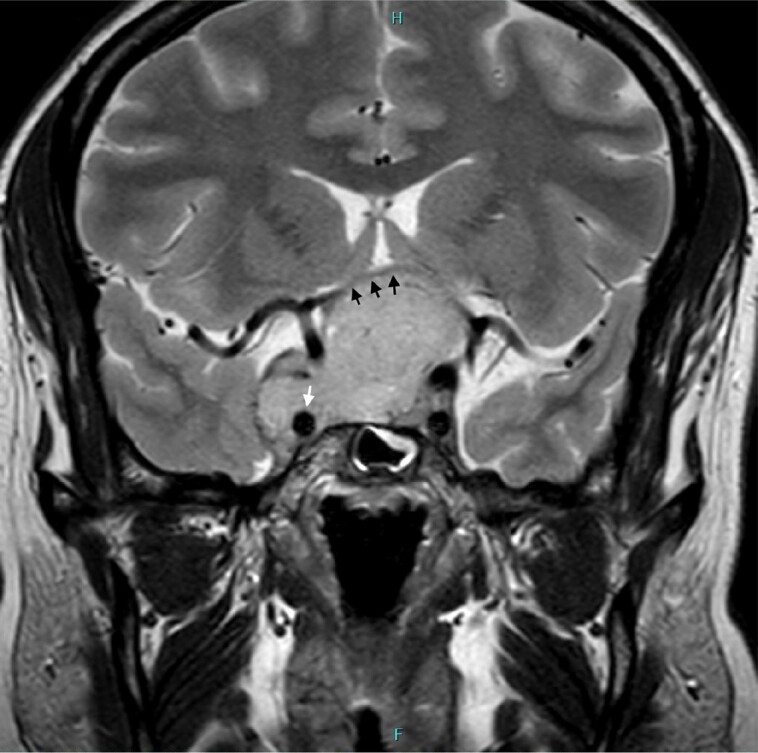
Coronal T2-weighted image again shows the sellar neoplasm with marked mass effect and thinning of the optic chiasm (arrowheads). There is near complete encasement of the right cavernous internal carotid artery (arrow).
